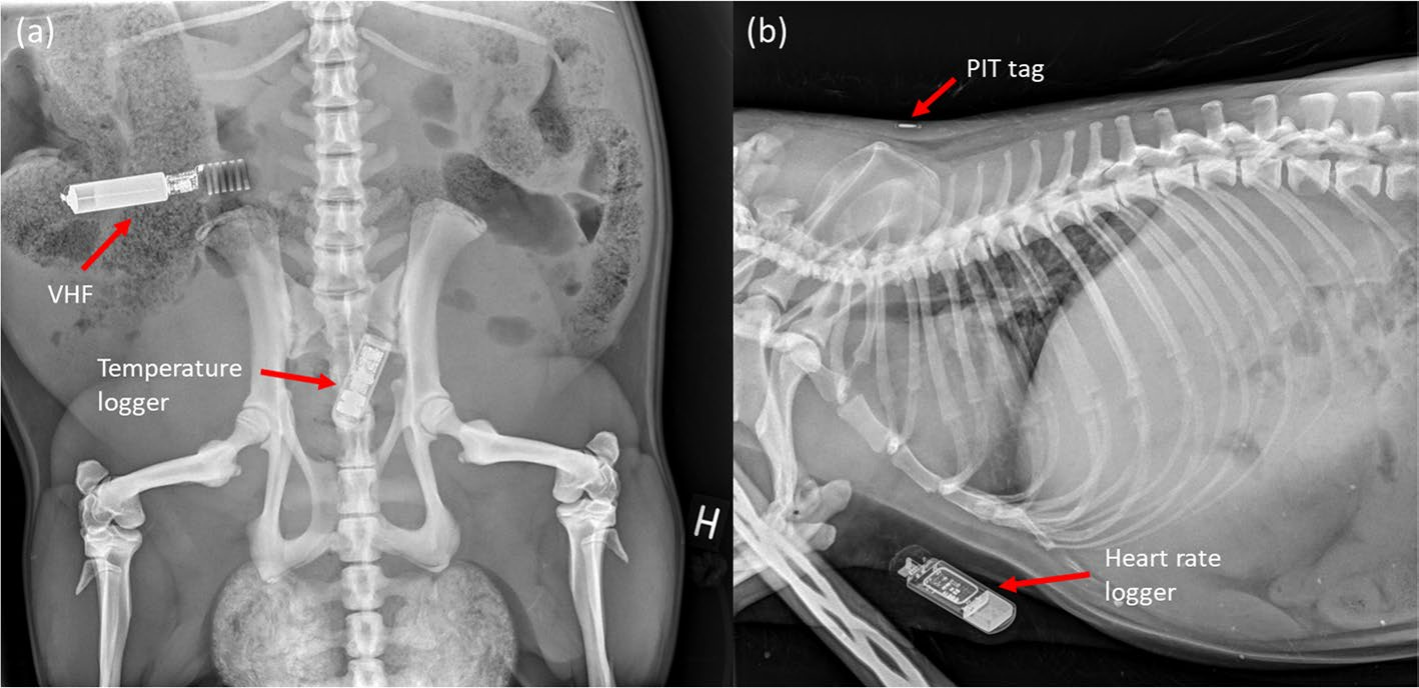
Dorsoventral and right lateral radiographs of two Eurasian beavers (*Castor fiber*) demonstrating the location of (a) a temperature logger (center) and VHF (top left) within the peritoneal cavity, and (b) a subcutaneous heart rate logger (bottom) and a subcutaneous PIT tag (top)
